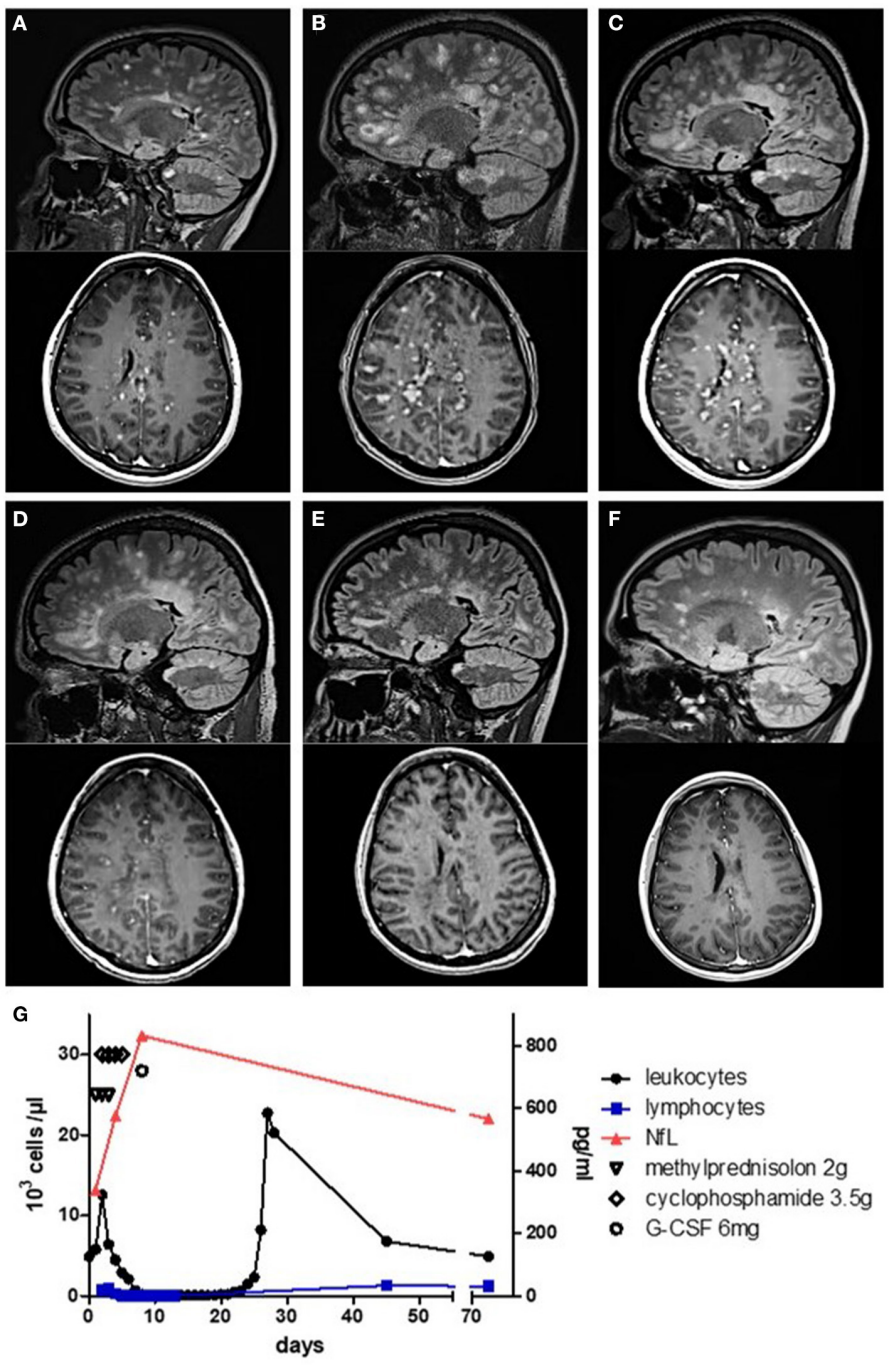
MRI and serological monitoring of the disease course. (A–F) Sagittal FLAIR and axial T1 gadolinium (Gad) sequences. (A) Numerous lesions with Gad enhancement at first admission. (B) MRI at re-admission showing more than 100 Gad-enhancing lesions. (C) Decrease of lesion load 7 days after the first dose of cyclophosphamide. (D) Further decrease of lesion load mirroring clinical remission at 15 days and (E) at 30 days. (F) At 44 days after cyclophosphamide treatment, no more Gad enhancement was detectable. (G) Leukocyte and lymphocyte count as well as serological neurofilament light chain levels during treatment.
